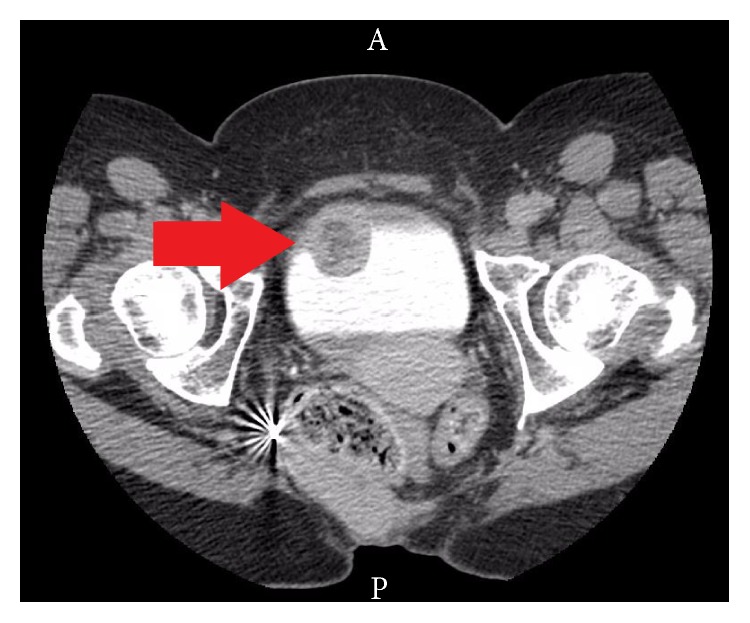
CT with contrast of the abdomen and pelvis demonstrating 3.2 cm anterior bladder mass (red arrow). The mass is a smooth, well-circumscribed lesion, which would be atypical for urothelial cell carcinoma.
